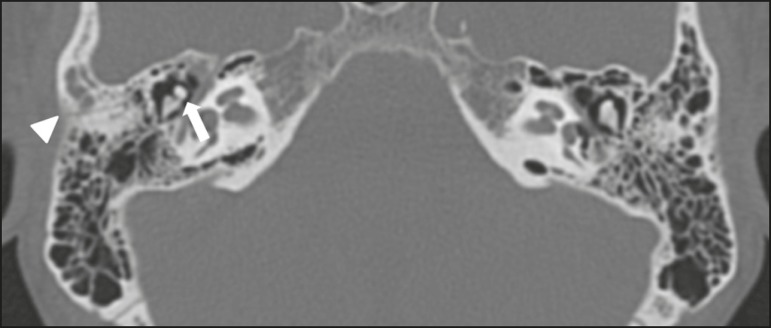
Fracture. Axial CT scan, with bone window settings, showing a
